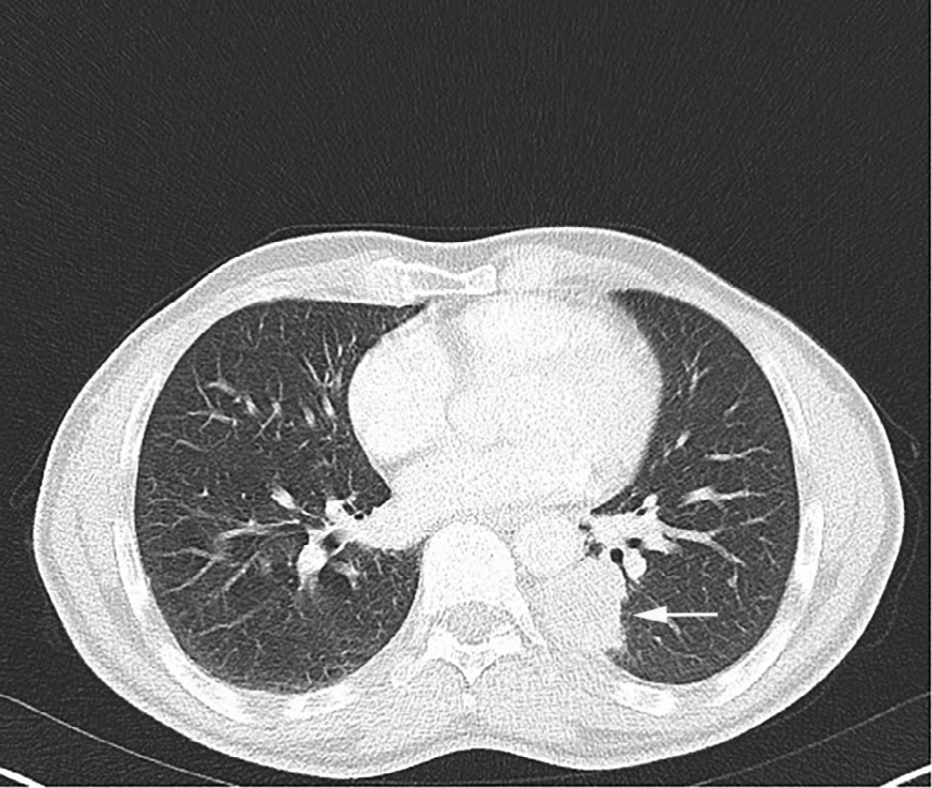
Chest computed tomography (CT) revealed progression of the tumor in the left lung (white arrow).
